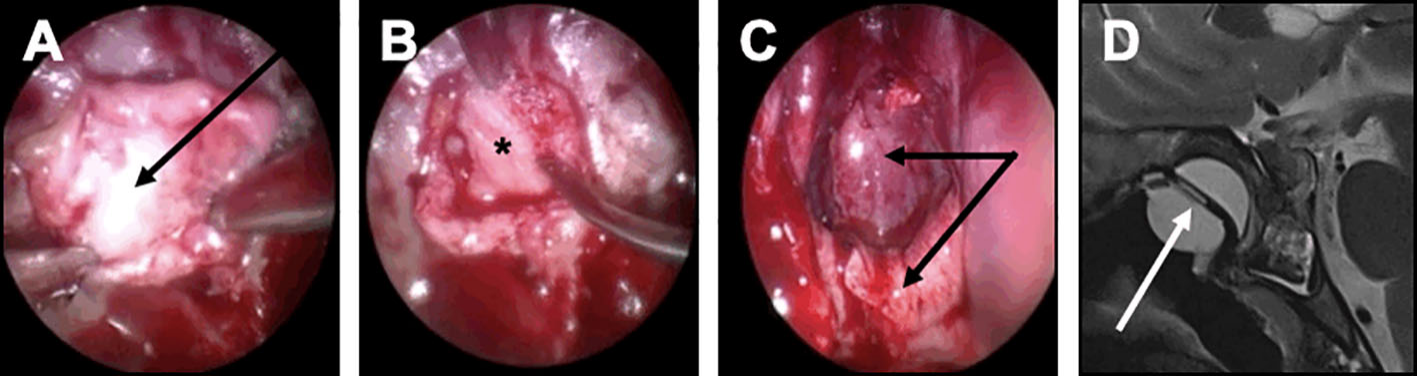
Closure strategy in patients with a risk factor. For these patients, a Foley urinary catheter is usually necessary and more complex multilayer closure strategies using a pedicled mucosal nasoseptal flap should be discussed. (A-D) Complex multilayer closure strategy for a patient with a recurrent pituitary adenoma treated with multiple surgery and radiotherapy. (A) After a muscle graft has been introduced in the intrasellar compartment, an autologous fascia lata graft has been positioned anterior to the sella turcica (black arrow). (B) After the fascia lata has been embedded in the epidural space with a bone graft (black asterisk), the primary sealing is obtained. (C) A vascularized mucosal nasoseptal flap is positioned and deployed in the sphenoid sinus (double black arrow), maintained using a Foley urinary catheter for 5 days. (D) Postoperative MRI showing the multilayer reconstruction and the Foley catheter (white arrow).
